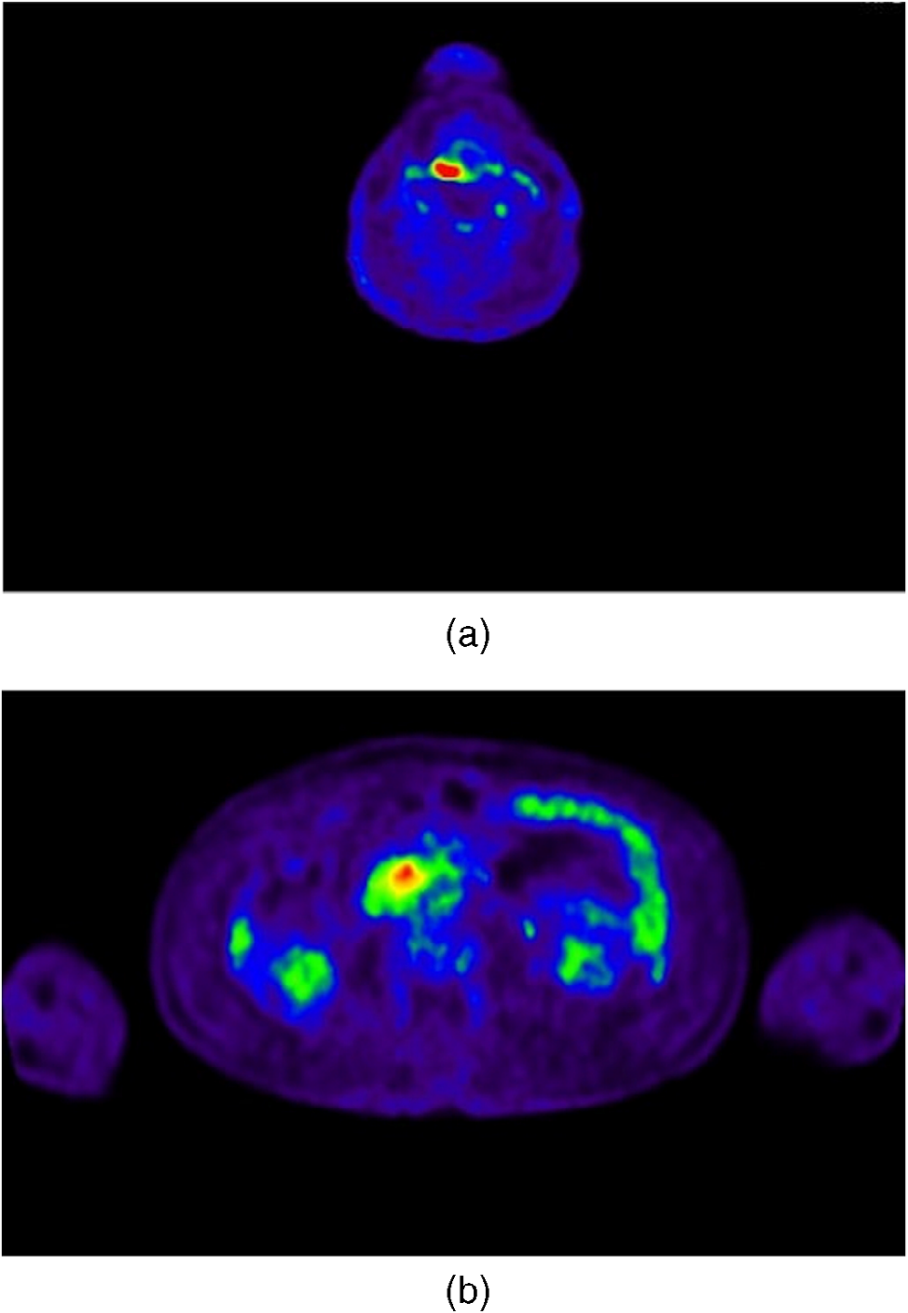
(a) Fluorodeoxyglucose hyperaccumulation in the right neck (SUVmax = 9.29). (b) FDG hyperaccumulation in the duodenum (SUVmax = 4.92).
